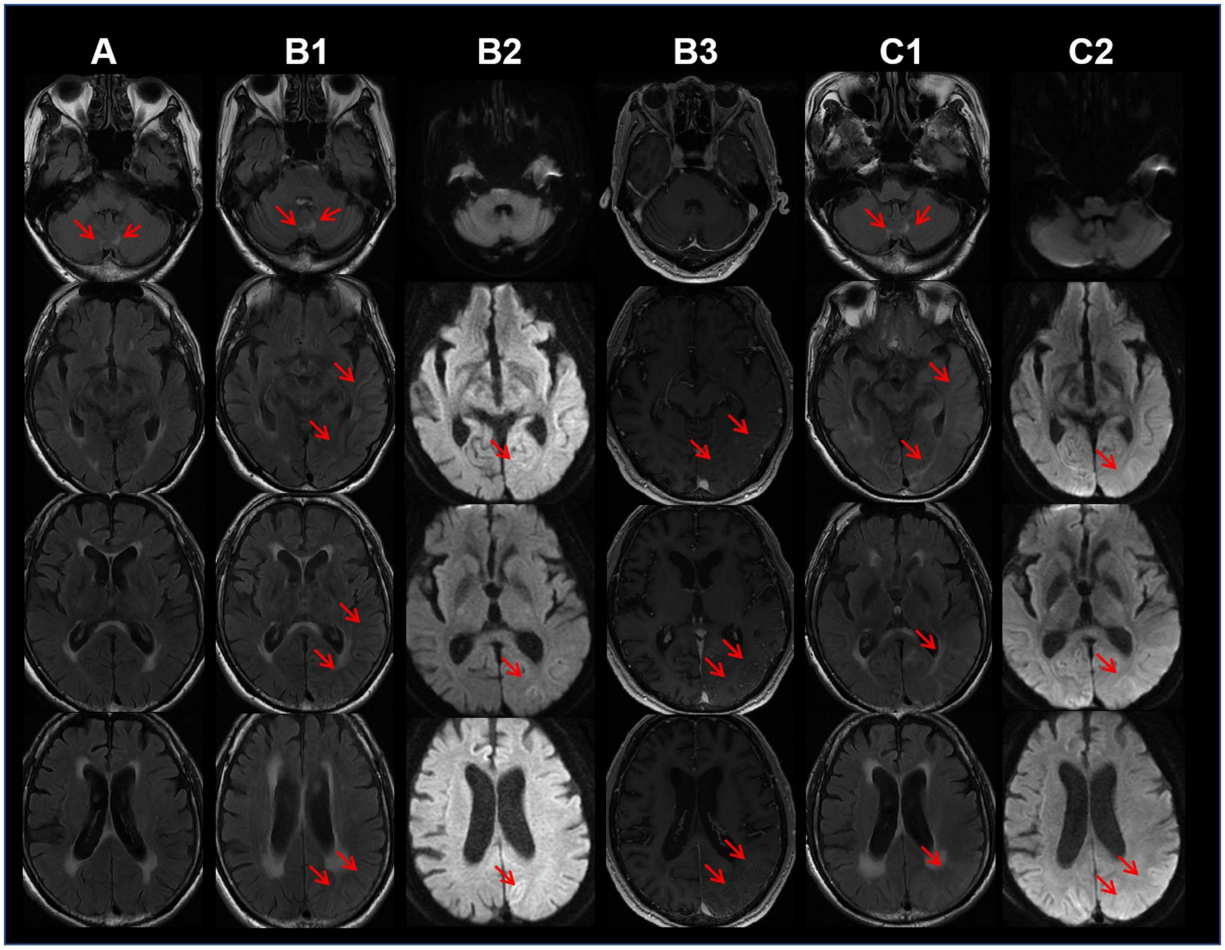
Column A: 22 months before dementia onset, the axial T2-weighted fluid attenuated inversion recovery (FLAIR) MRI sequence shows bilateral hyperintensities in the cerebellar paravermal region. Column B: Ten days after dementia onset, FLAIR sequence (B1) shows edema in the temporo-occipital lobes, diffusion-weighted imaging (DWI) (B2) reveals mildly restricted diffusion in the lesions, and contrast-enhanced MRI (B3) shows mild enhancement of the lesions. Column C: One month follow-up after discharge (52 days after symptom onset), FLAIR sequence (C1) demonstrates cortical edema and subcortical white matter hyperintensities, while DWI (C2) shows mildly restricted diffusion in the lesions.
